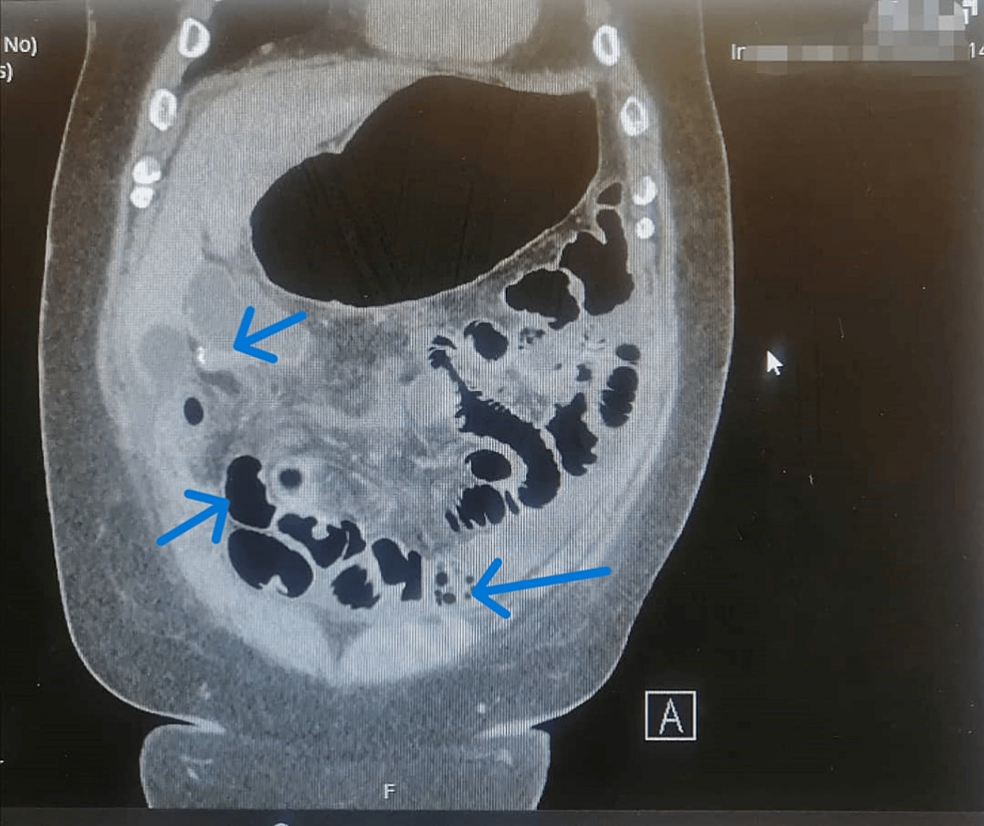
CT of the abdomen and pelvis with oral and IV contrast on POD 5 showing a dilated small intestine with no transitional point. The colon contains gas extending to the rectum, suggesting ileus rather than obstruction, and there is no evidence of a leak. POD: Postoperative day.
